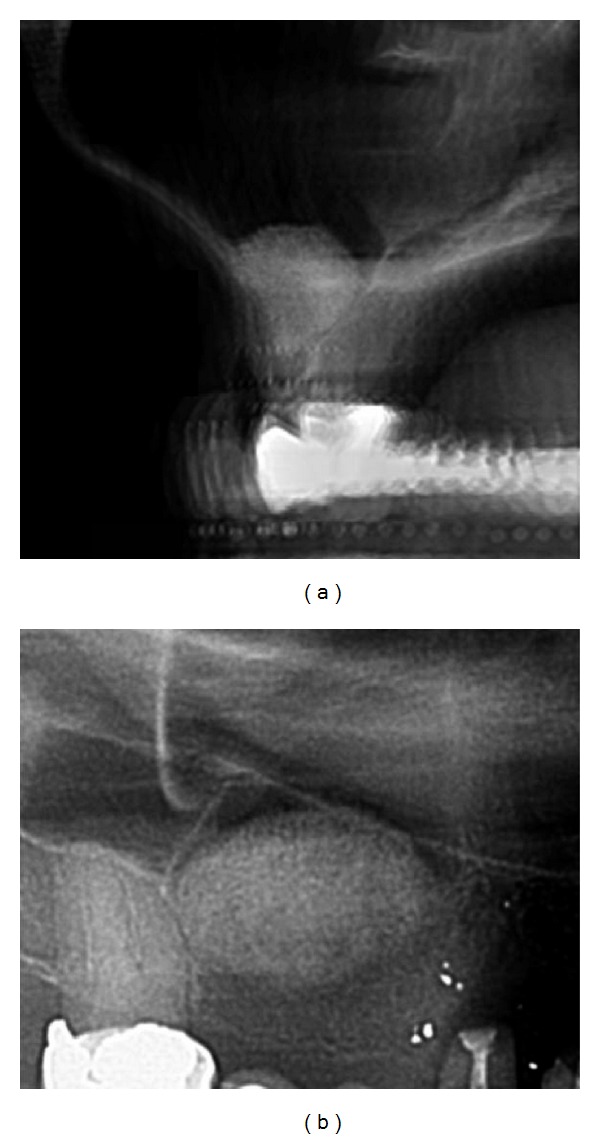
Case 3: transversal and parasagittal CBCT scan 7 months following tHUCSL-Intralift. The ossification process is obviously completed. A slim denser line on the antral floor marks the transition to the original alveolar crest.
